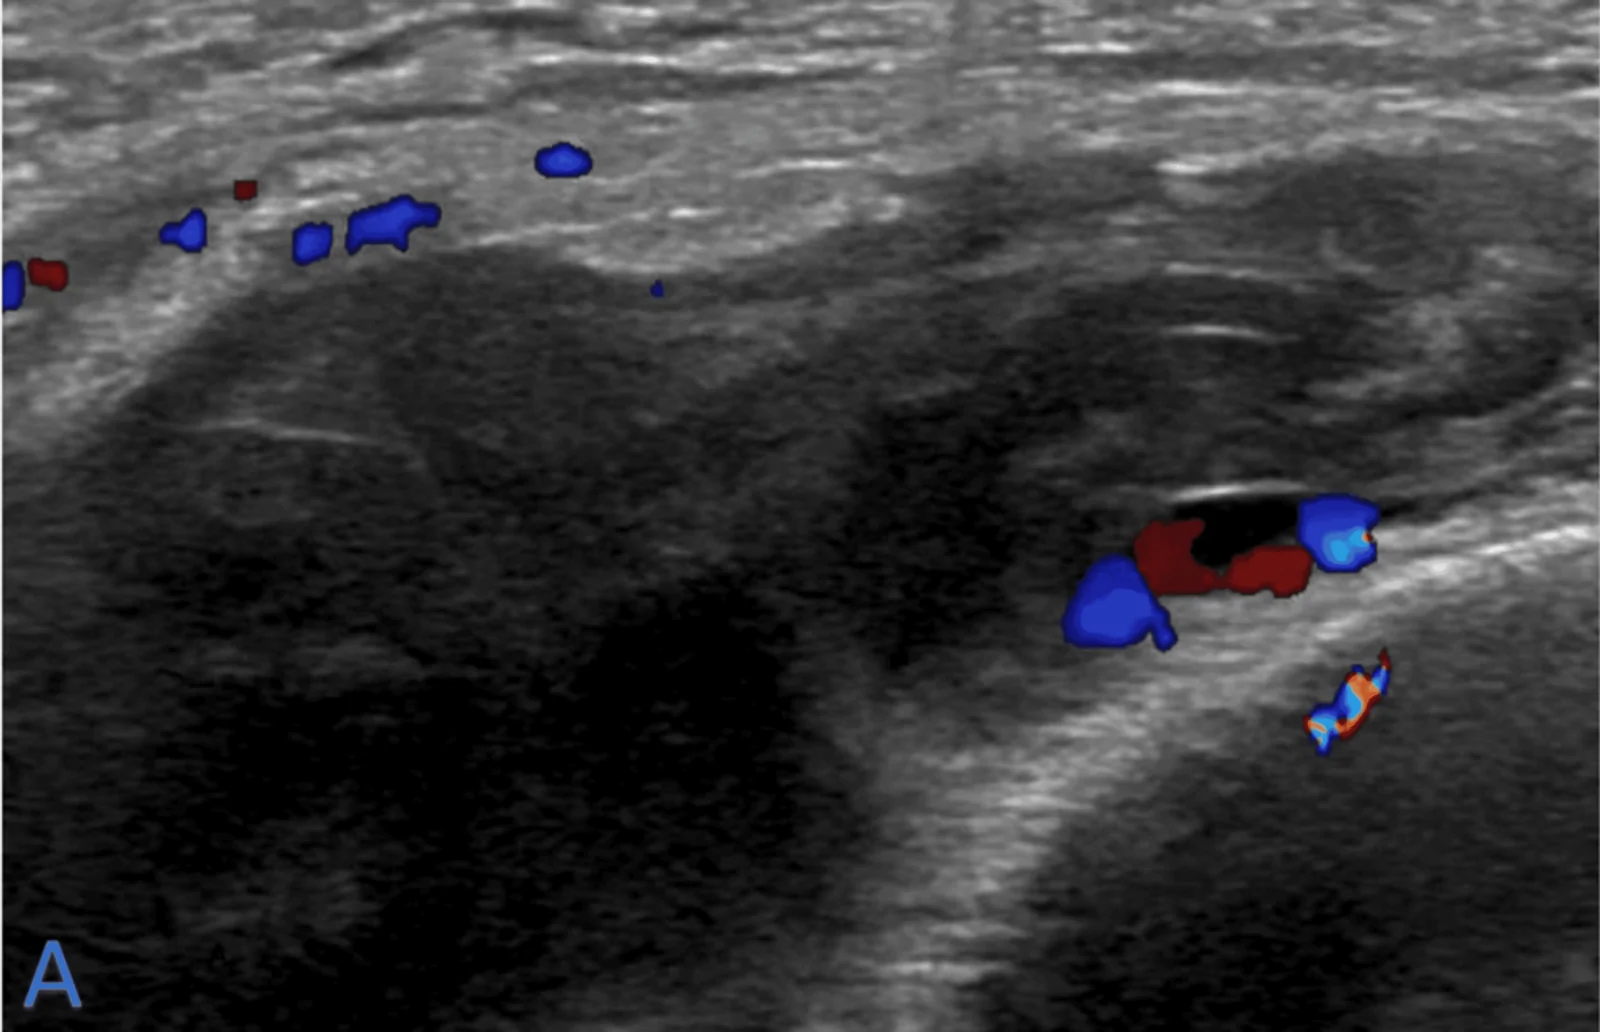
Follow-up ultrasound image of the knee Transverse follow-up ultrasound image showing a fairly heterogeneous subcutaneous collection at the medial aspect of the distal right thigh with a small linear vascular structure.
